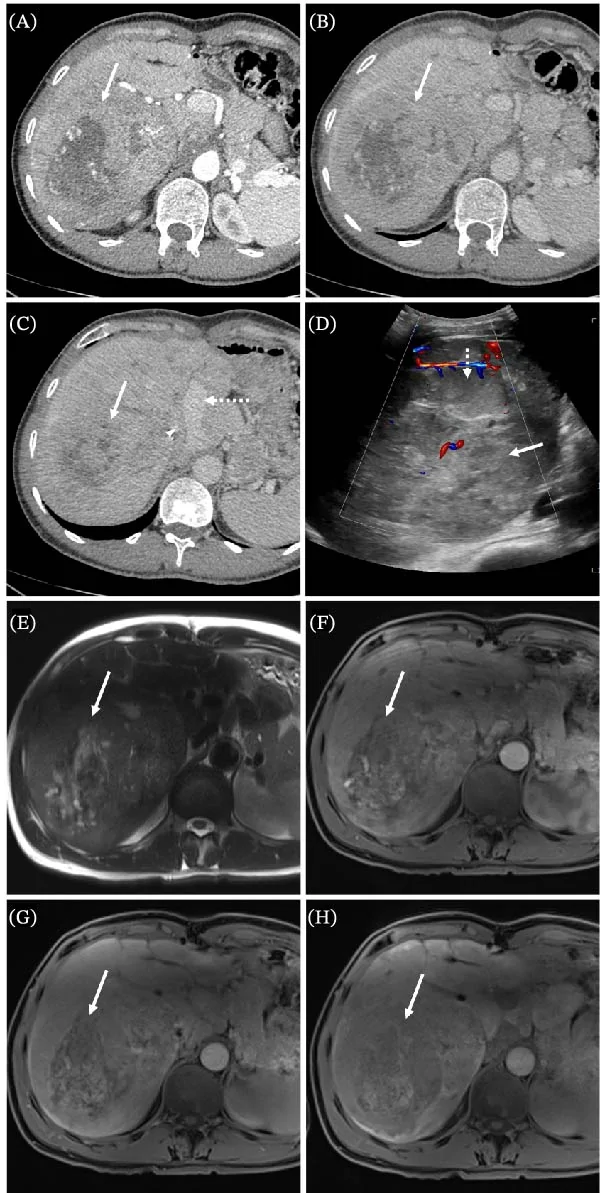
(A–C) Axial contrast‐enhanced CT scan of the abdomen in the arterial phase (A) and portal venous phase (B, C) demonstrates a large heterogeneously enhancing mass in the right hepatic lobe (arrow) with central necrosis in both the arterial and portal venous phases. A portocaval shunt was also observed (arrowhead) (C). (D) Grayscale and color Doppler ultrasound image of the right hepatic lobe showed a large heterogeneous mass (arrow) with prominent internal vascularity and feeding vessels (dotted arrow). (E–H) The axial T2‐weighted MR image (E) shows a heterogeneous mass with predominantly intermediate signal intensity and central T2 hyperintensity corresponding to areas of necrosis. Axial T1‐weighted MR images obtained with gadoxetic acid in the arterial phase (F), portal venous phase (G), and 20‐min hepatobiliary phase (H) show a heterogeneously enhancing mass with areas of arterial phase hyperenhancement and washout in the portal venous phase, and a hypointense signal relative to the background liver in the hepatobiliary phase.
